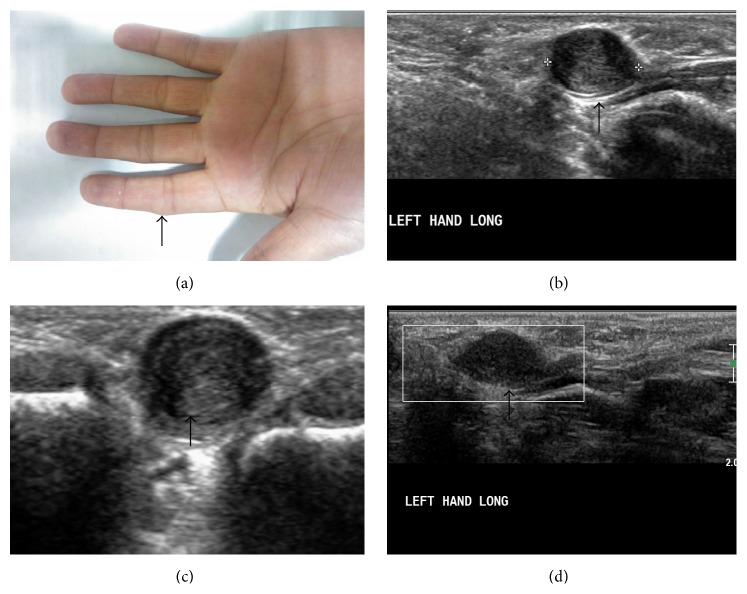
Schwannoma. A 25-year-old female patient presented with firm, noncompressible swelling over the radial aspect of the proximal interphalangeal joint of 2nd digit ((a), arrow). Ultrasonography ((b)–(d)) showed a well-defined, oval, hypoechoic, hypovascular lesion (arrow).
